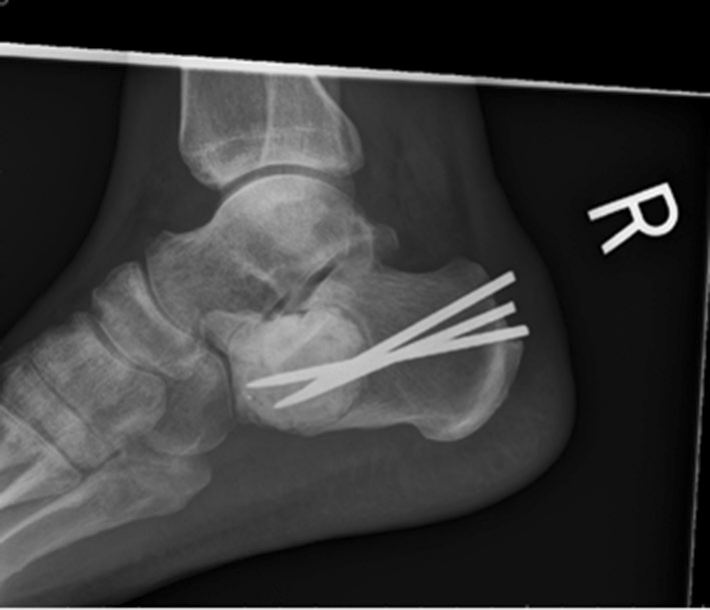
Two-year post-operative follow-up radiograph of the calcaneus showing curettage and cementing with Steinman pin reinforcement. There has been so sign of recurrence of disease or failure of the construct despite full activity
